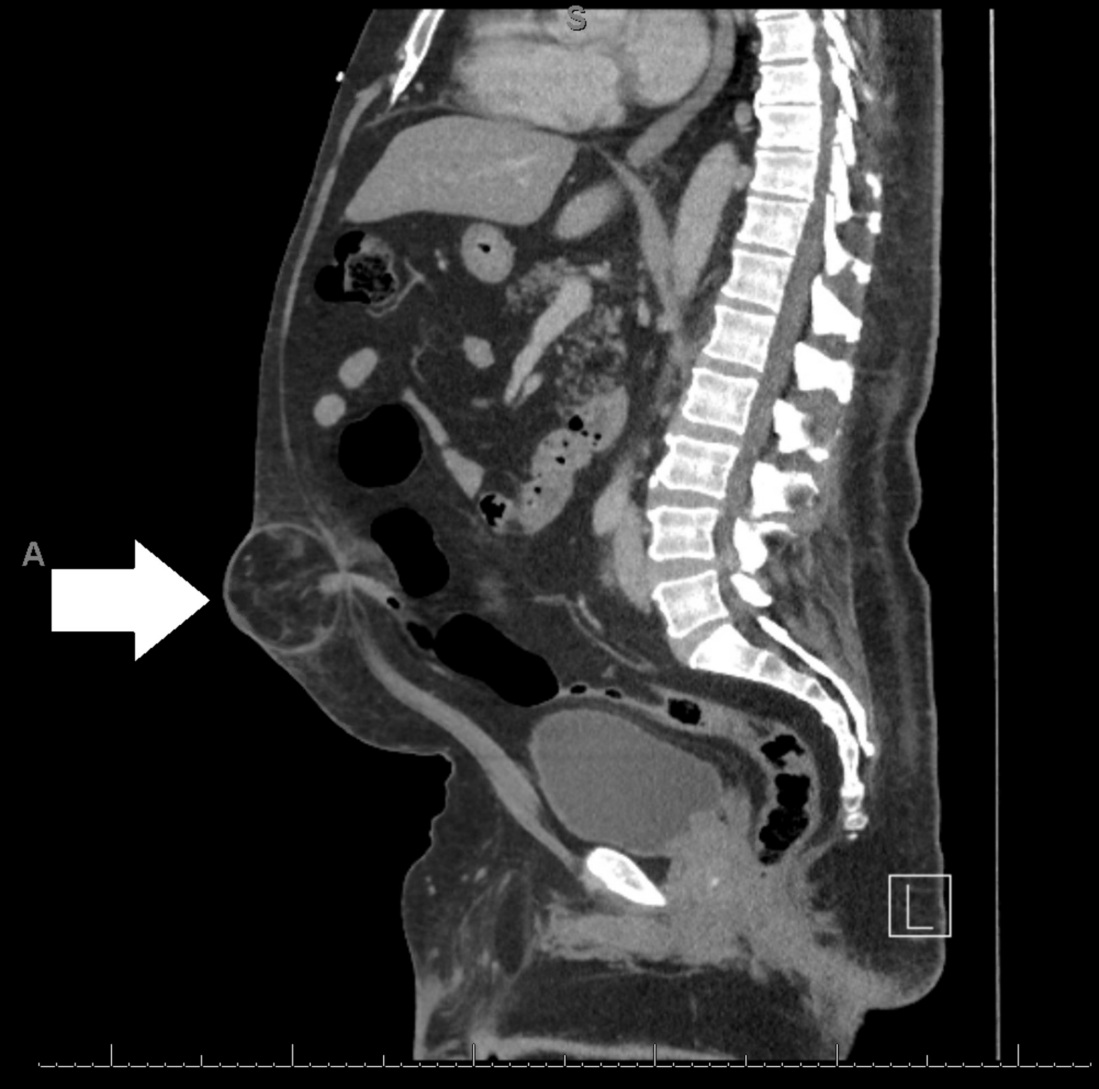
Sagittal CT showing umbilical hernia containing the appendix.
